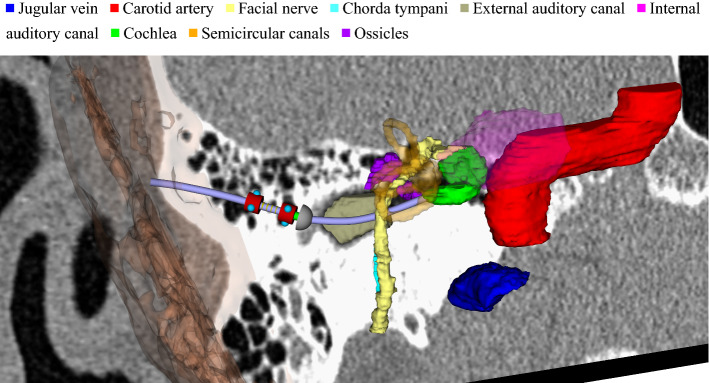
Robotic drilling of a nonlinear access canal through the temporal bone requires preoperative planning consisting of two steps: segmentation of risk structures within the temporal bone (white bone on the CT slice) and trajectory planning for a collision-free trajectory from the surface of the skull (transparent) to the clinical target (e.g., the cochlea)
